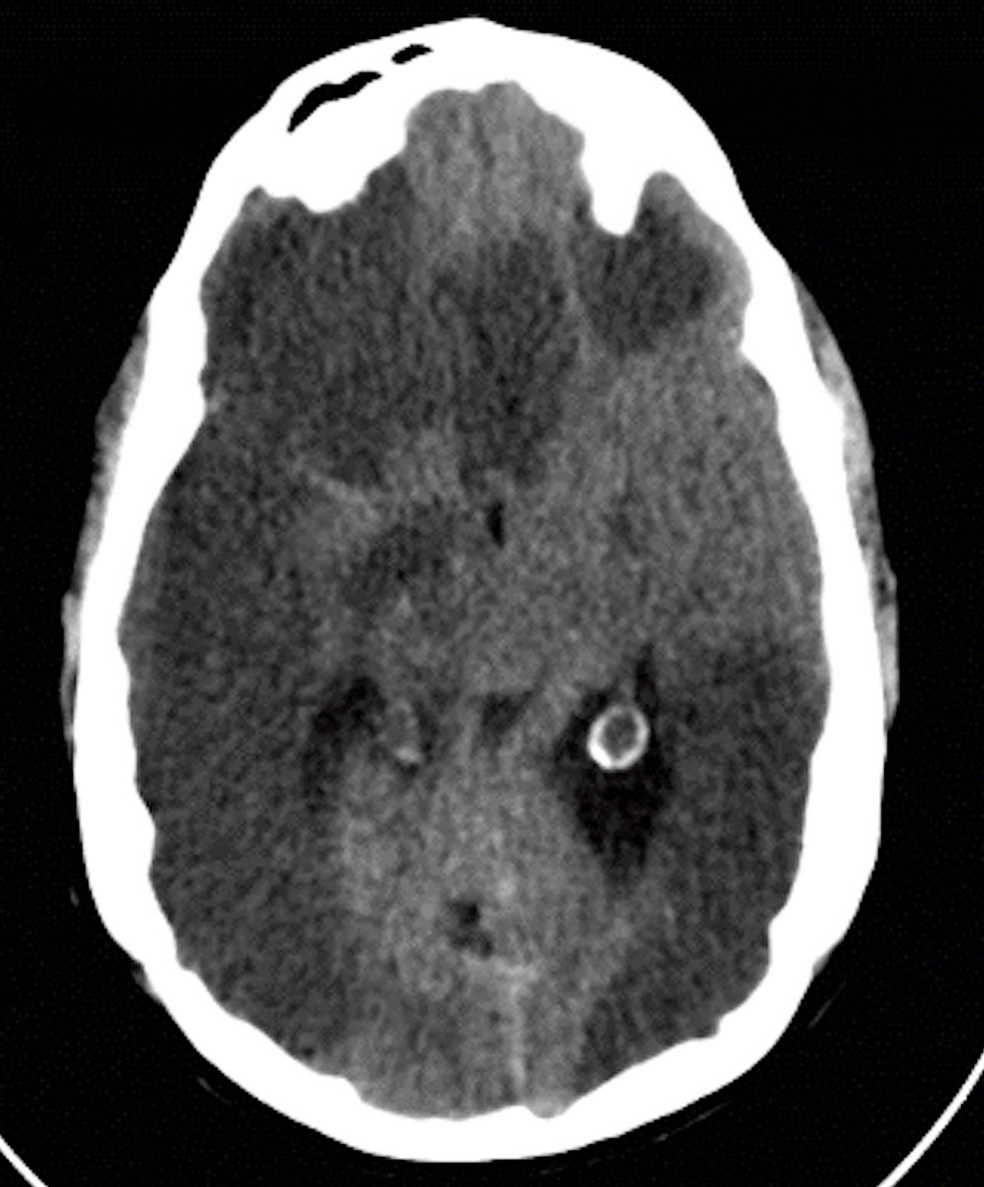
CT of the brain showing multiple hypodense regions representing established areas of brain infarction
